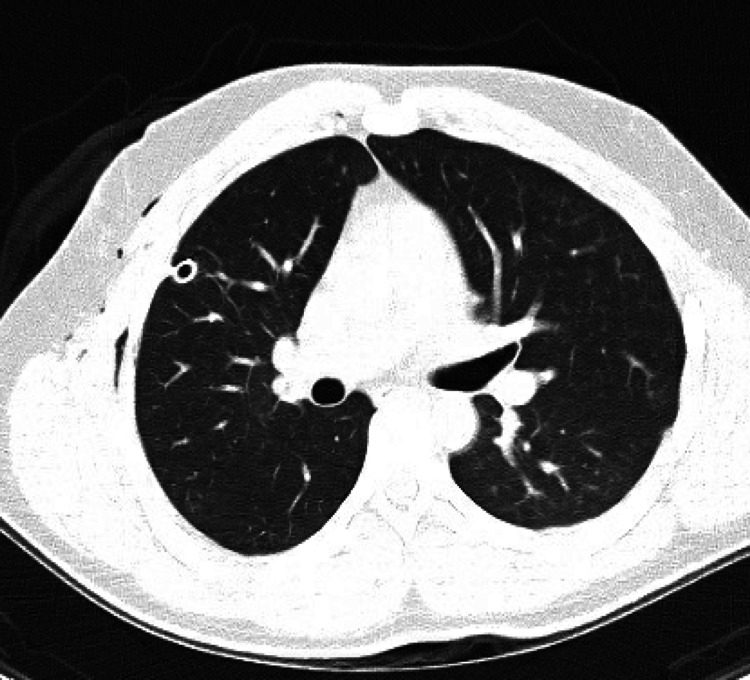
Computed tomography showing subcutaneous emphysema in the right chest wall
